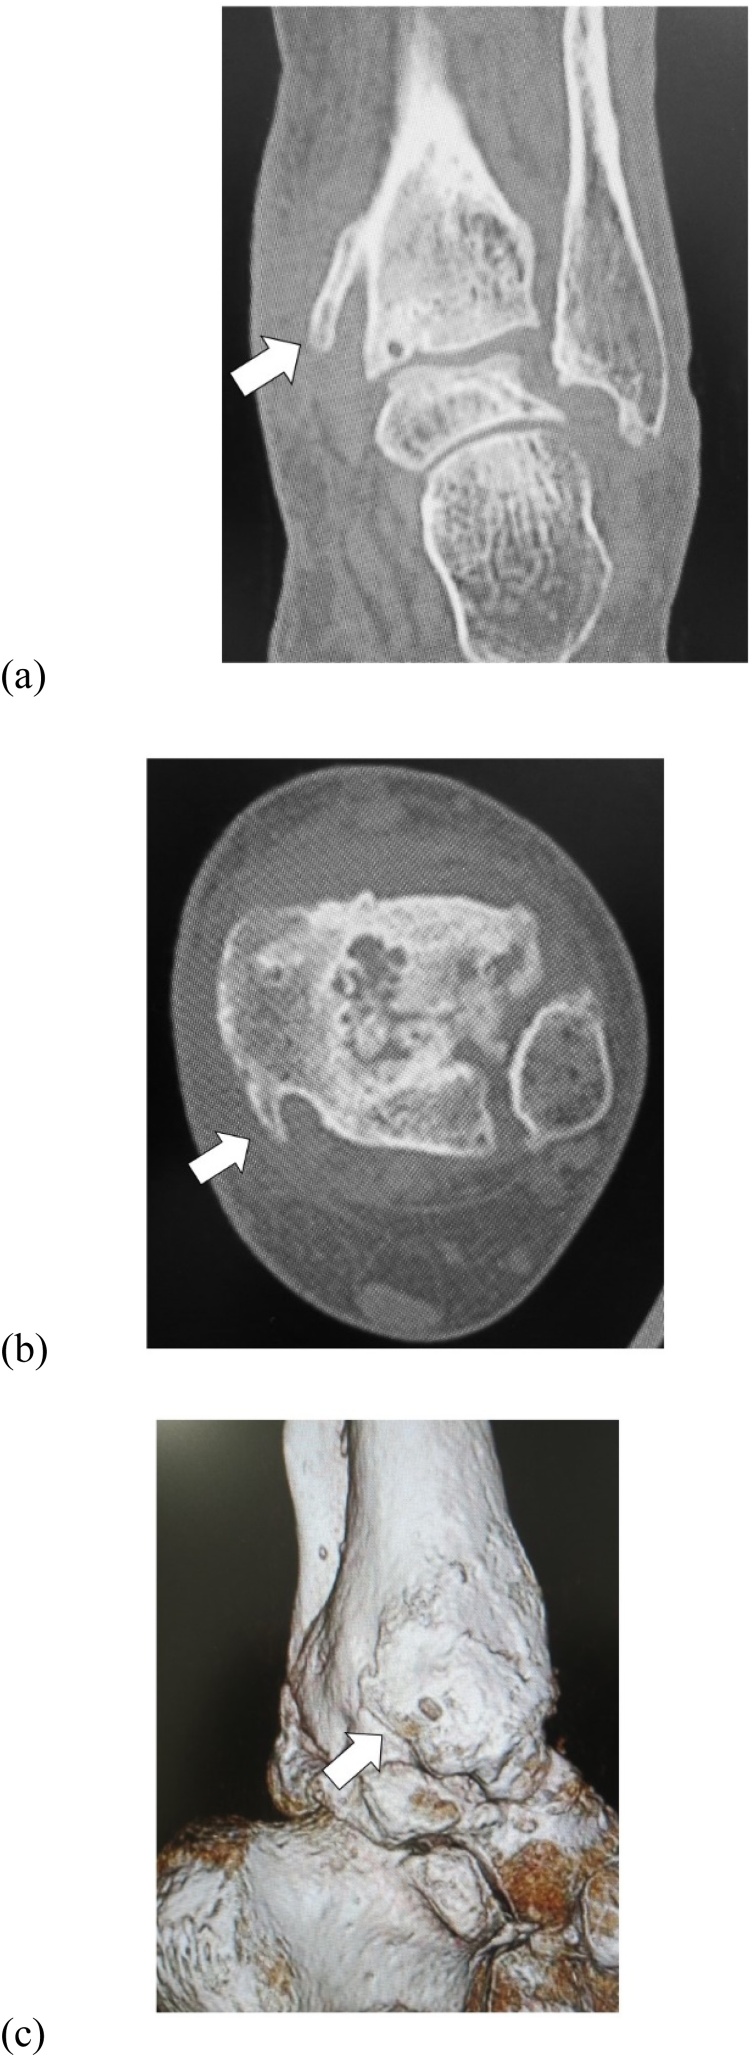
Preoperative computed tomography (CT) images. CT shows osteophyte of the posteromedial aspect of the distal tibia (arrow) on (a) coronal view and (b) axial view and on (c) 3D CT imaging.
